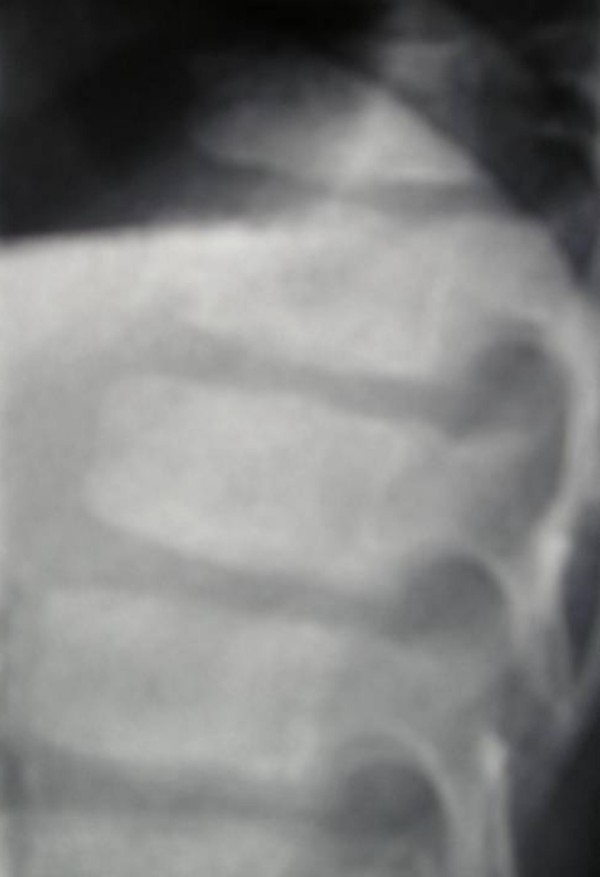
Lateral spine radiograph showed platyspondyly associated with anterior clefting of the vertebrae.
